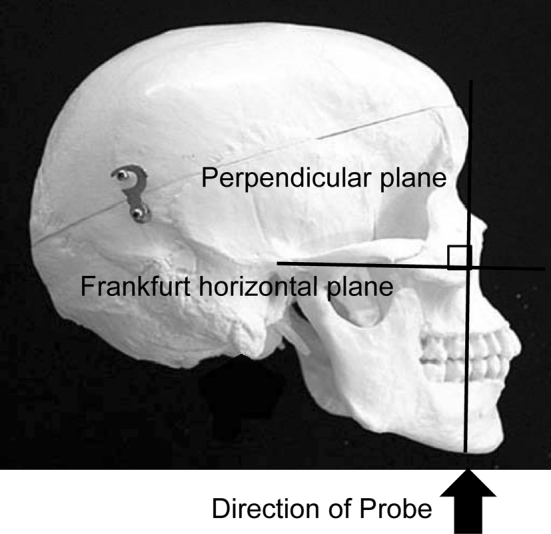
Position of ultrasonic probe in lateral view. The measurement points were determined at the center of the plane perpendicular to the Frankfurt horizontal plane in a frontal section. The perpendicular plane passes through the distal surfaces of the mandibular second premolars on both sides
